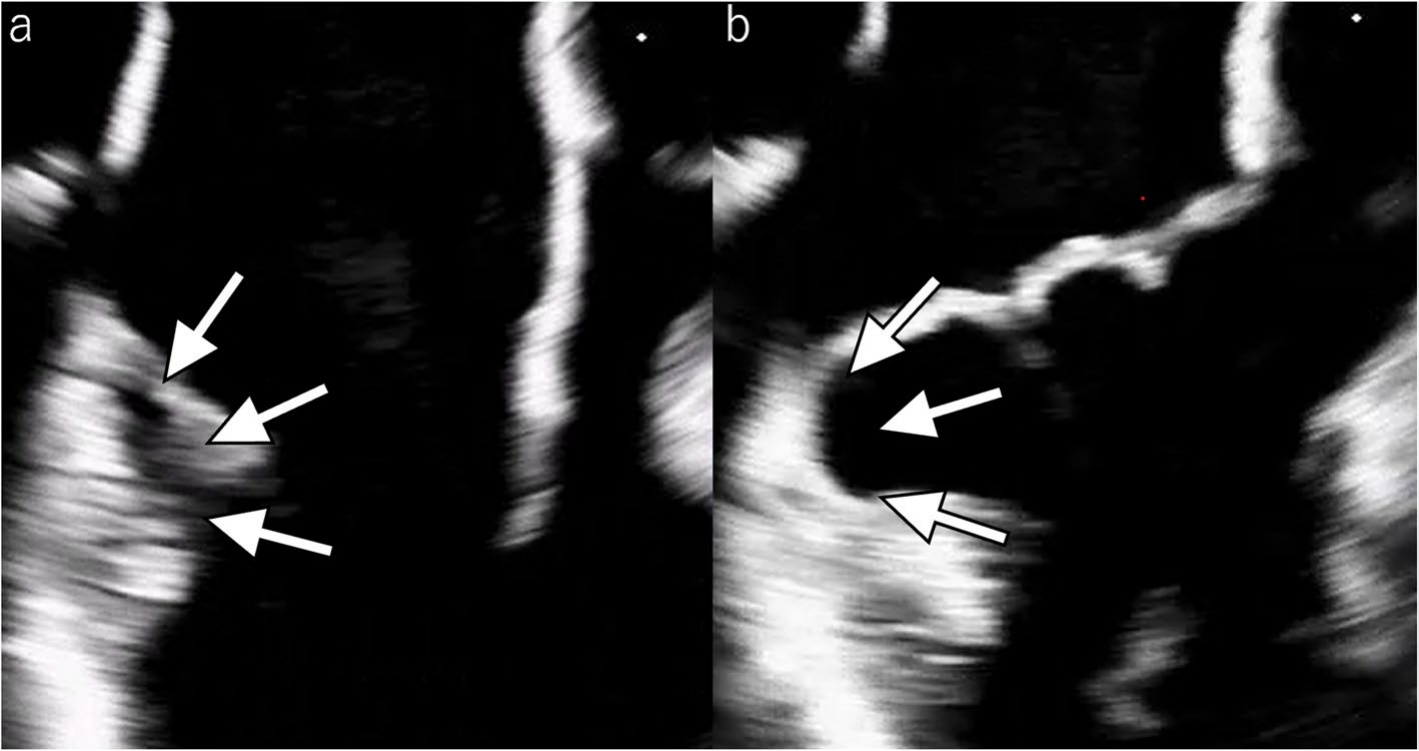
Preoperative transesophageal echocardiographic images (a diastole, b systole). The arrows indicate the areas of mitral annular disjunction
